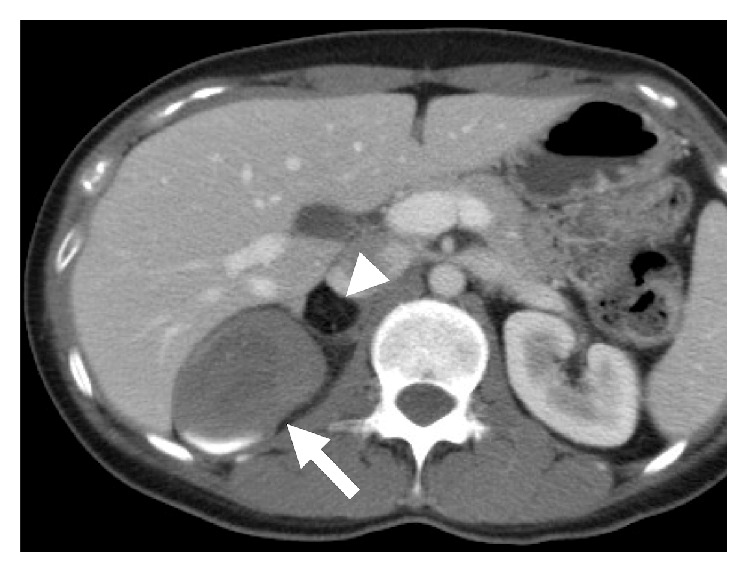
Axial contrast-enhanced CT scan. The mass consisted of a cystic component (arrow) and a nodular fatty component (arrowhead) at the right adrenal region. A high-density fluid-fluid level was also seen in the cystic component. No enhancing component was observed.
